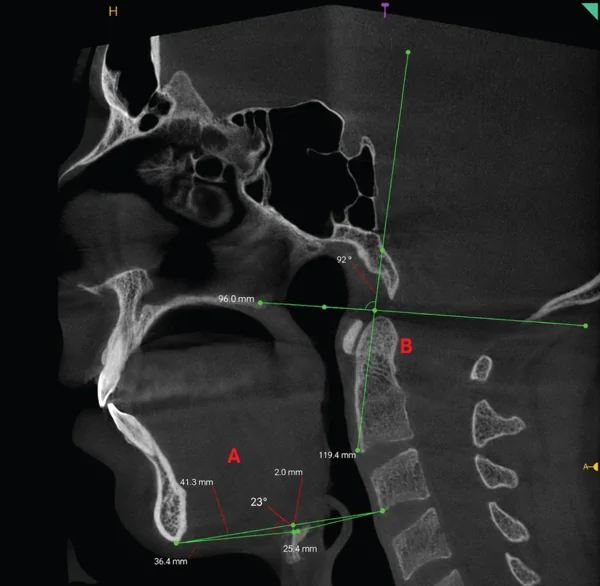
Case 2 pretreatment CBCT reveals a narrow hypodivergent hyoid triangle. (A) A hypodivergent triangle placed with the hyoid bone below the level of C3 with an angulation of 23 degrees. (B) Cervical vertebrae alignment.
